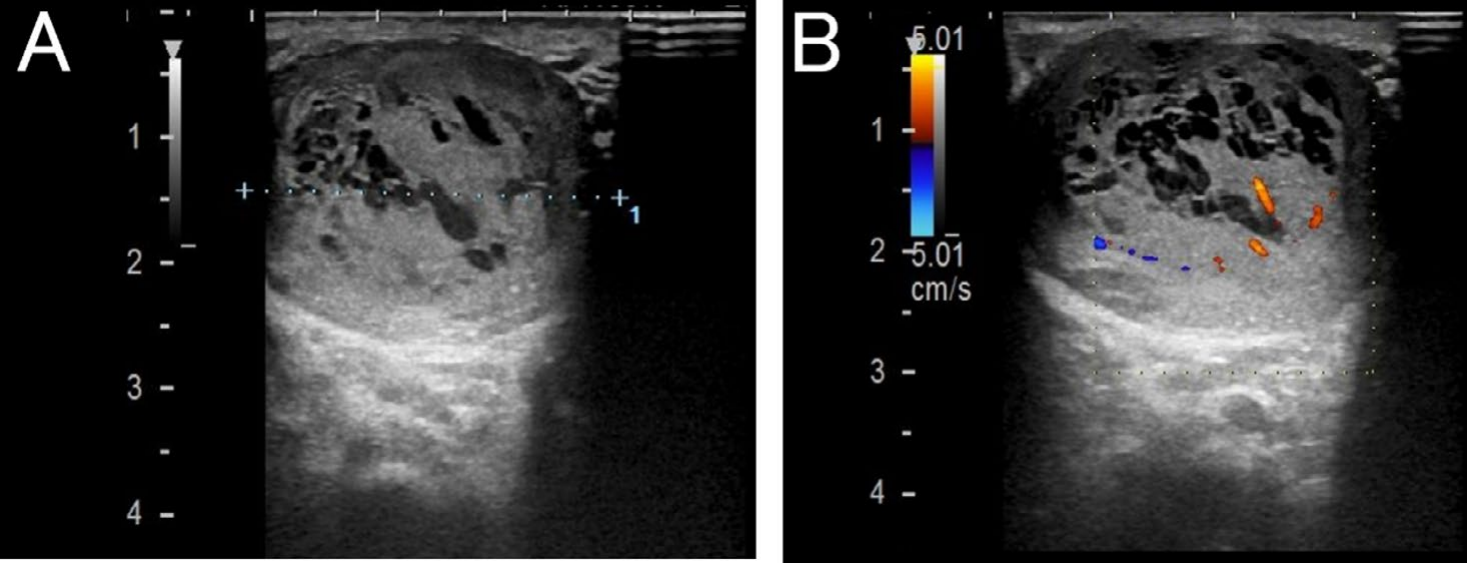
Ultrasonographic findings of the left testis. (A) The tumor occupies most of the left testis measuring 3 × 4.4 × 2.6 cm in size. Tumor showed heterogeneous echogenicity, and has some anechoic cystic lesions. (B) Color Doppler showed blood flow inside the tumor.
